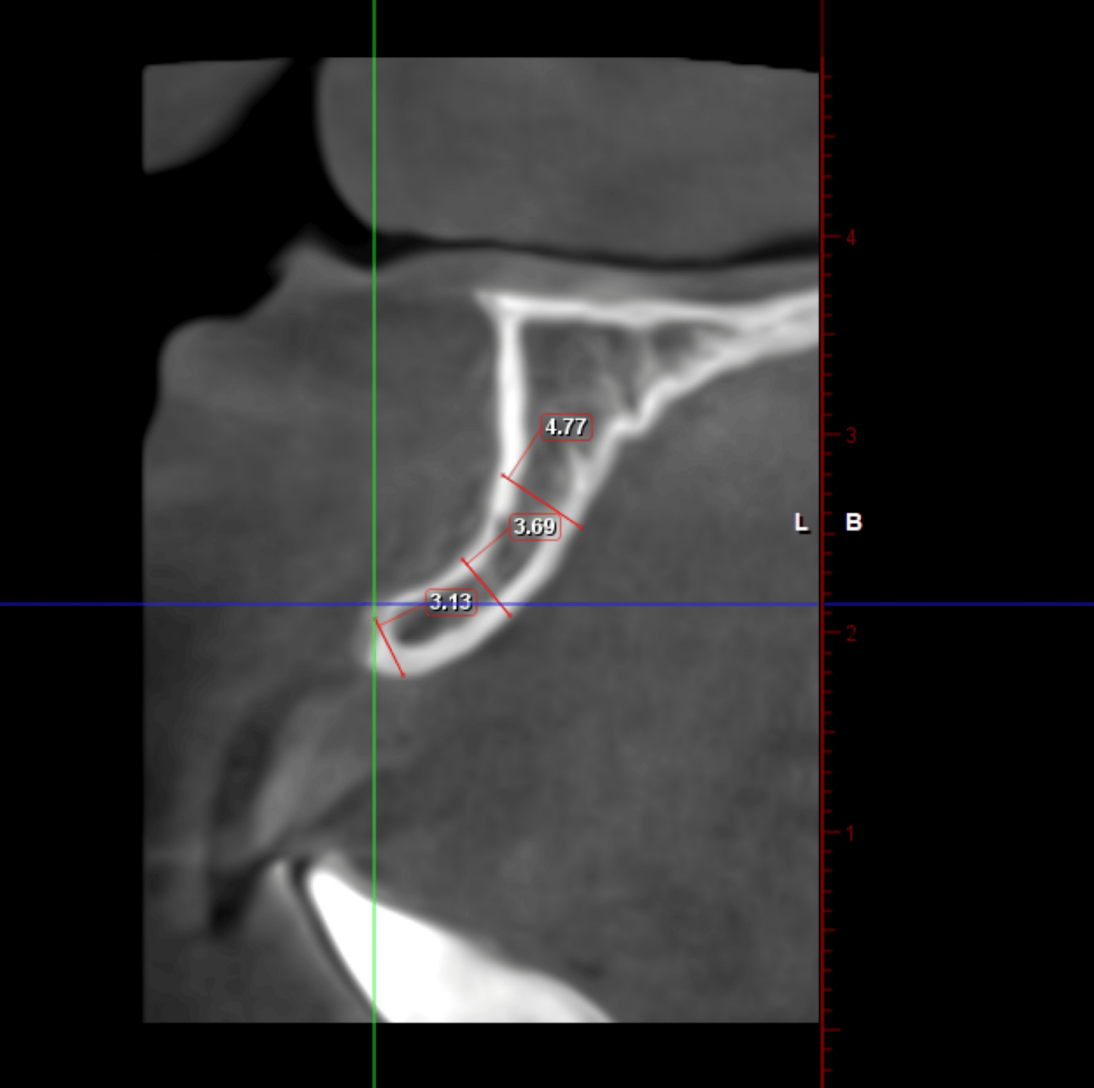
Case 1: Coronal section of CBCT image showing 3.13 mm of buccolingual bone at the crest, 3.69 mm bone at the middle third, and 4.77 mm bone apically denoting inadequate buccolingual bone for implant placement using conventional osteotomy CBCT: Cone beam computed tomography.
